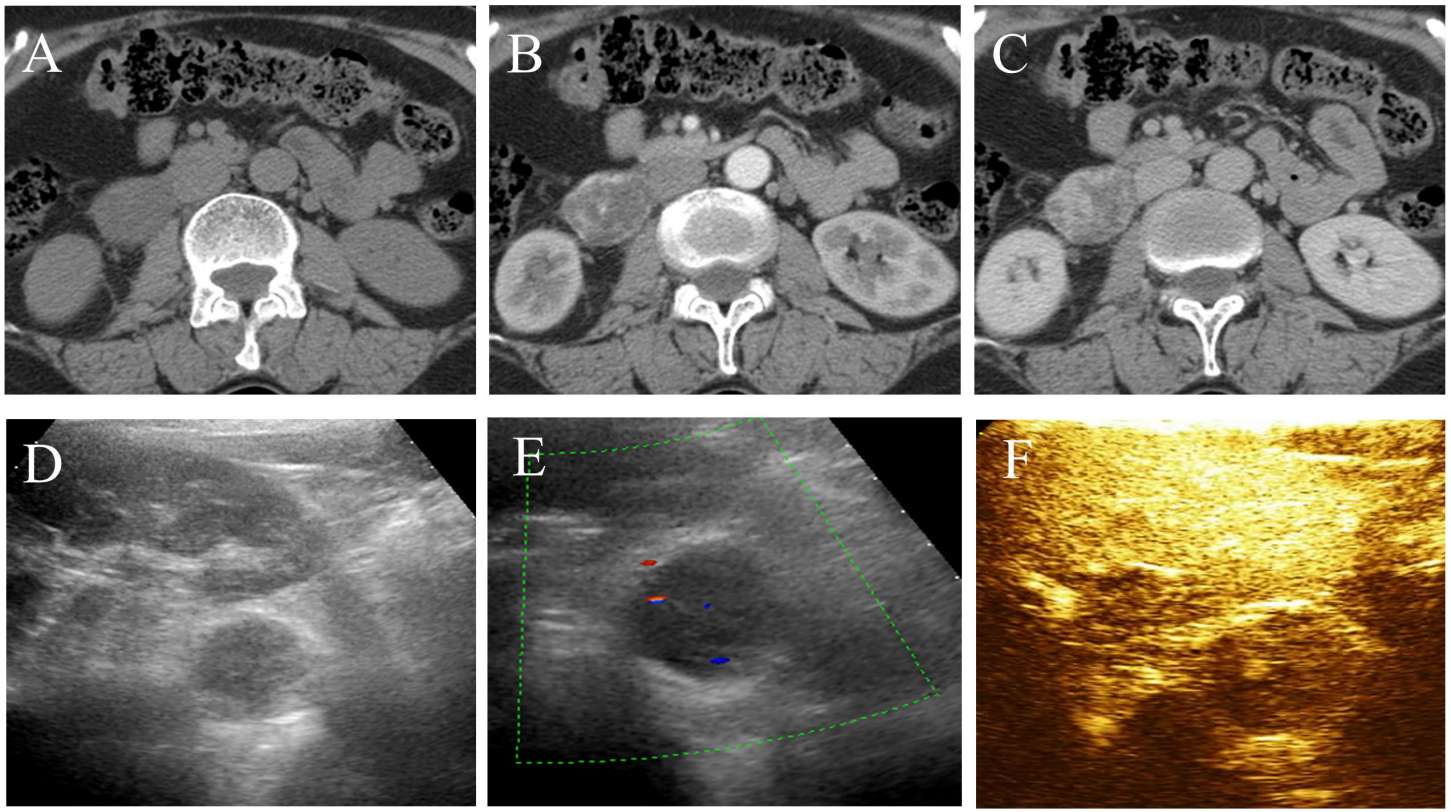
CT and ultrasound features of retroperitoneal anastomosing hemangioma. (A) Plain scan. (B) Arterial phase. (C) Venous phase. (D, E) The lesion exhibits a hypoechoic mass with slight blood flow signals in the inferior anteromedial region of the right kidney. (F) Contrast-enhanced ultrasound demonstrates homogeneous and “slow-in and slow-out” low enhancement.
